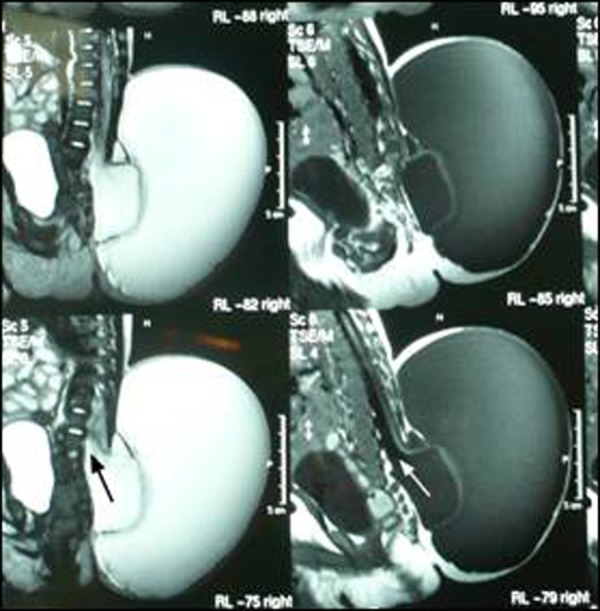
Figure 2: MRI sagittal section showing two cysts. The inner cyst was in continuation (arrow) with the central canal of the spinal cord
